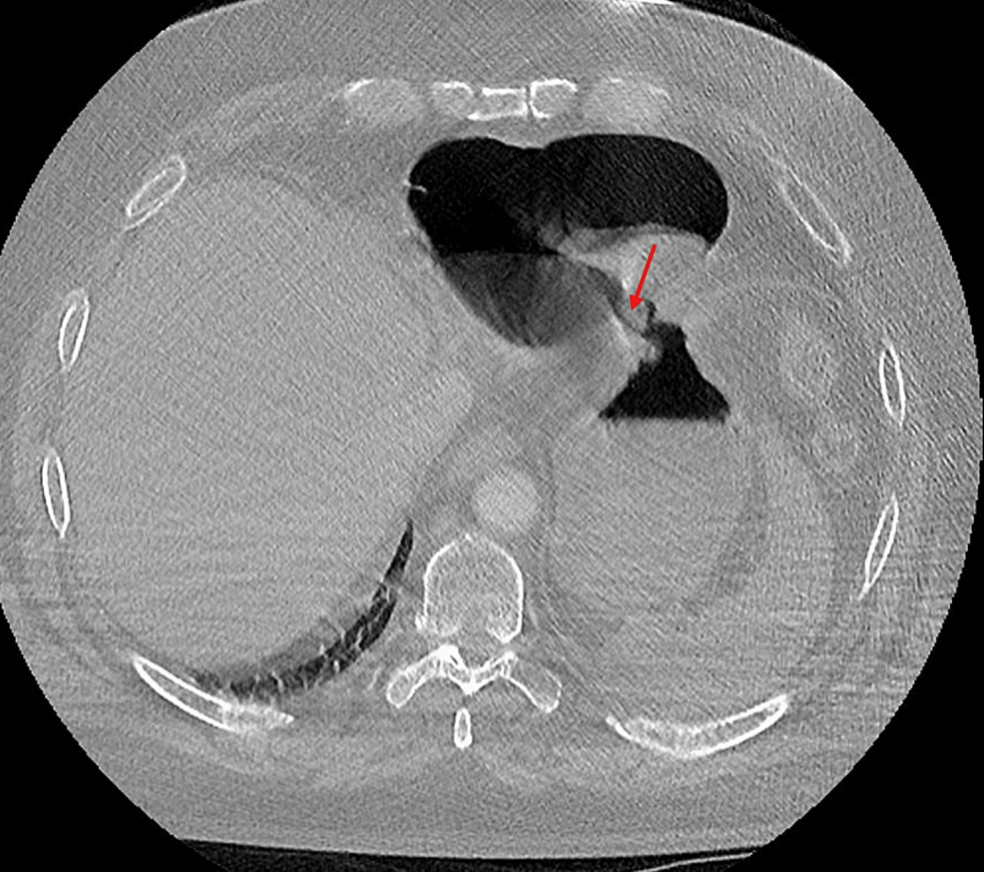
CT scan showing a connection between the stomach and the pericardium (arrow referring to the gastro-pericardial fistula)
